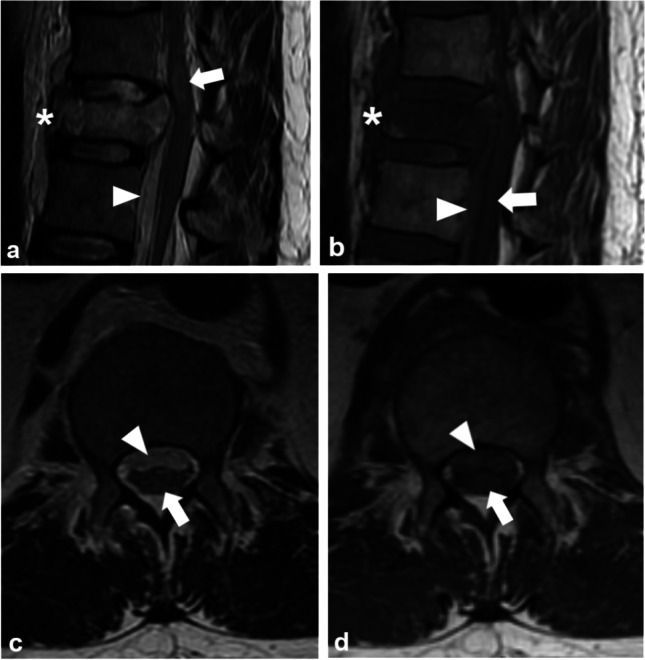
A 66-year-old patient fell three meters off a ladder on his stomach and was unable to move his lower limbs. A sagittal T2WI from an MRI scan revealed a spinal cord injury (arrow) caused by a burst fracture of Th12 (asterisk). Anteriorly to the spinal cord is a hyperintense spinal hematoma (arrowhead) visible in the subdural space (a). On sagittal T1WI, the subdural hematoma (arrowhead) appears hyperintense compared to the spinal cord (arrow). The burst fracture is shown as a low-intensity area on T1WI representing multiple fracture lines and oedema across the vertebral body (asterisk) (b). On transverse T2WI, the subdural hematoma (arrowhead) is contained within the dural sac and pressed against the spinal cord (arrow) (c). On transverse T1WI, the subdural hematoma (arrowhead) shows a higher intensity compared to the posteriorly located spinal cord (arrow) (d). MRI = magnetic resonance imaging, Th12 = twelfth thoracic vertebra
